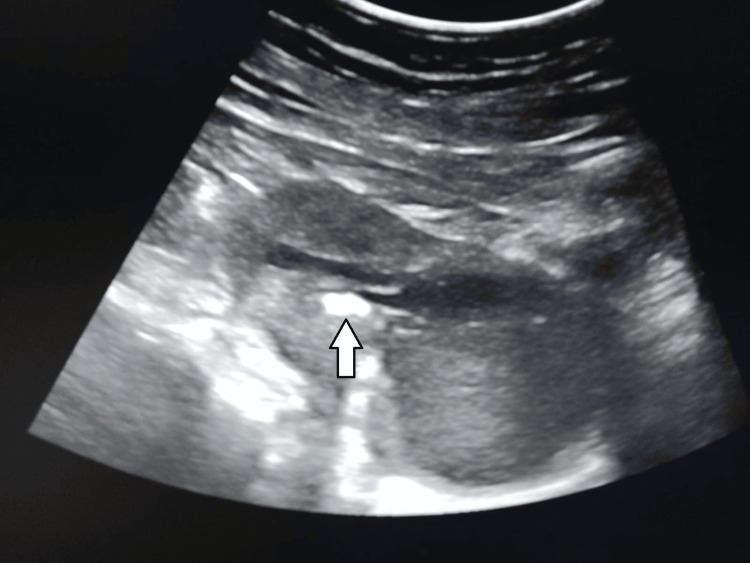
2D transvaginal ultrasound shows a large collection inside the uterine cavity with homogenous internal echoes and echogenic foci (white arrow).
